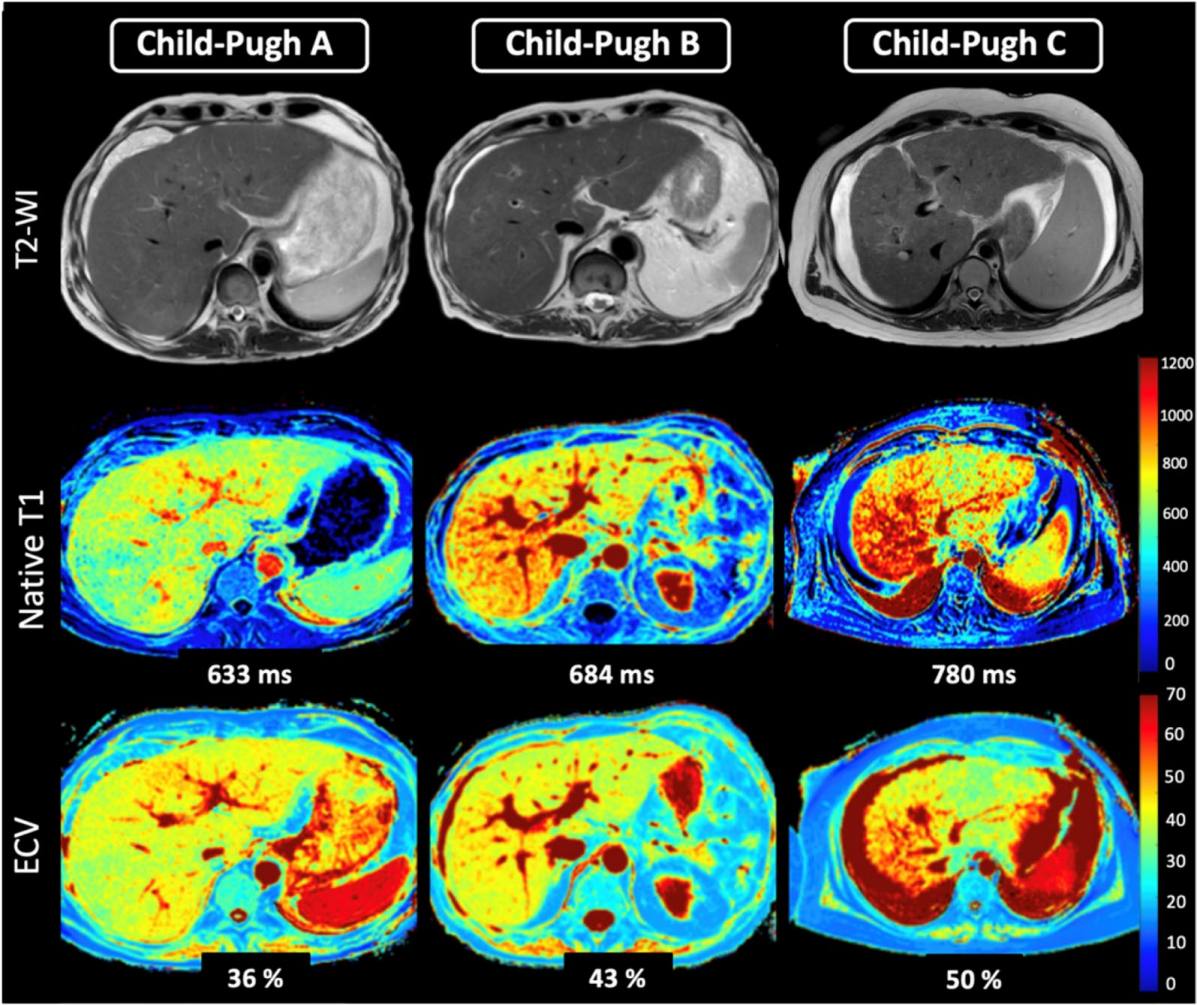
Representative images of T2-weighted images, hepatic native T1 and extracellular volume fraction (ECV) maps from a 54-years-old male patient with liver cirrhosis Child–Pugh class A, from a 61-year-old female patient with Child–Pugh class B, and a 41-year-old female patient with Child–Pugh class C. T1 relaxation times and ECV show increased values depending on Child–Pugh class. *T2-WI* T2-weighted image, *ECV* extracellular volume fraction.
